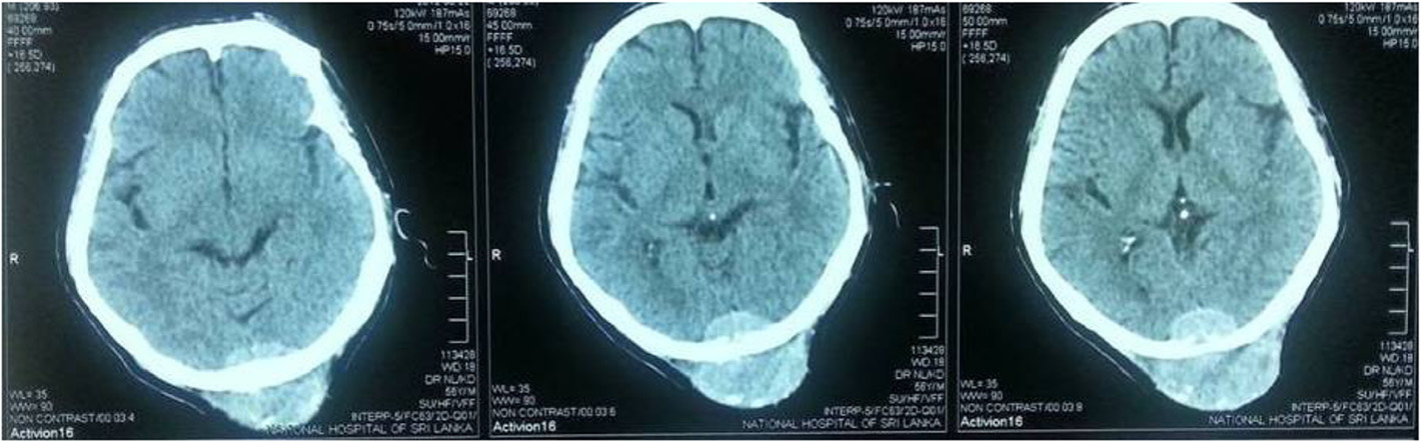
Contrast CT brain showing skull deposit with extra and intracranial extension
